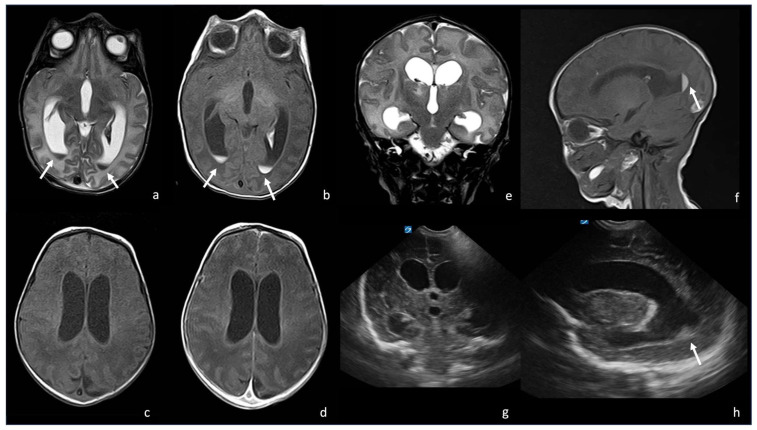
A 9-day-old boy affected by meningitis caused by *Listeria Monocytogenes* with ventriculitis. There are hypointense in T2 (arrow in (a), axial T2WI) and hyperintense detritus in T1 (arrow in (b), axial T1-weighted images); a slight contrast enhancement of ventricle wall is visible in (d), axial post- contrast T1 WI, especially if compared with (c) axial pre-contrast T1 WI. There is also a ventriculomegaly visible on both MRI ((e), coronal T2WI; (f), sagittal T1 WI) and US ((g), coronal plane and (h), parasagittal plane). In (f,h), there are also visible hyperintense ((f), arrow) and hyperechogenic detritus ((h), arrow).
